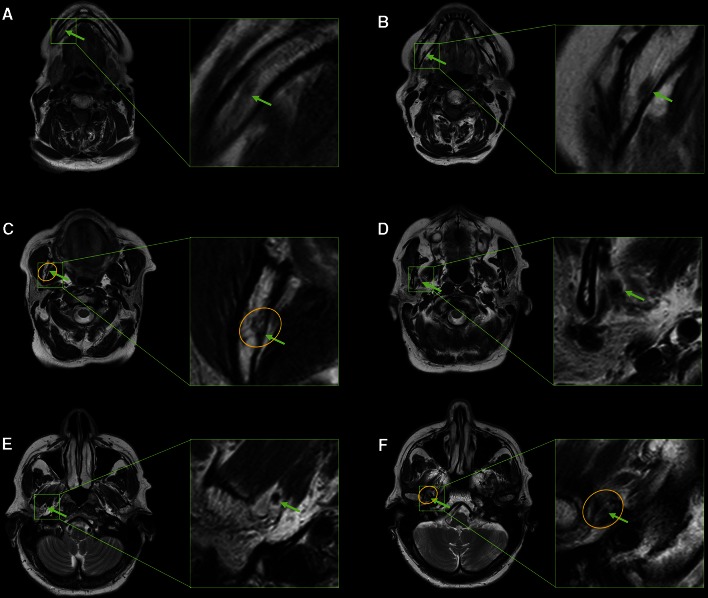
Serial axial 3T-MRI slices (T2w turbo spin-echo sequence) showing the course of the healthy IAN (*green arrows*) from the mandible (a) through the mandibular foramen (c, *yellow*
